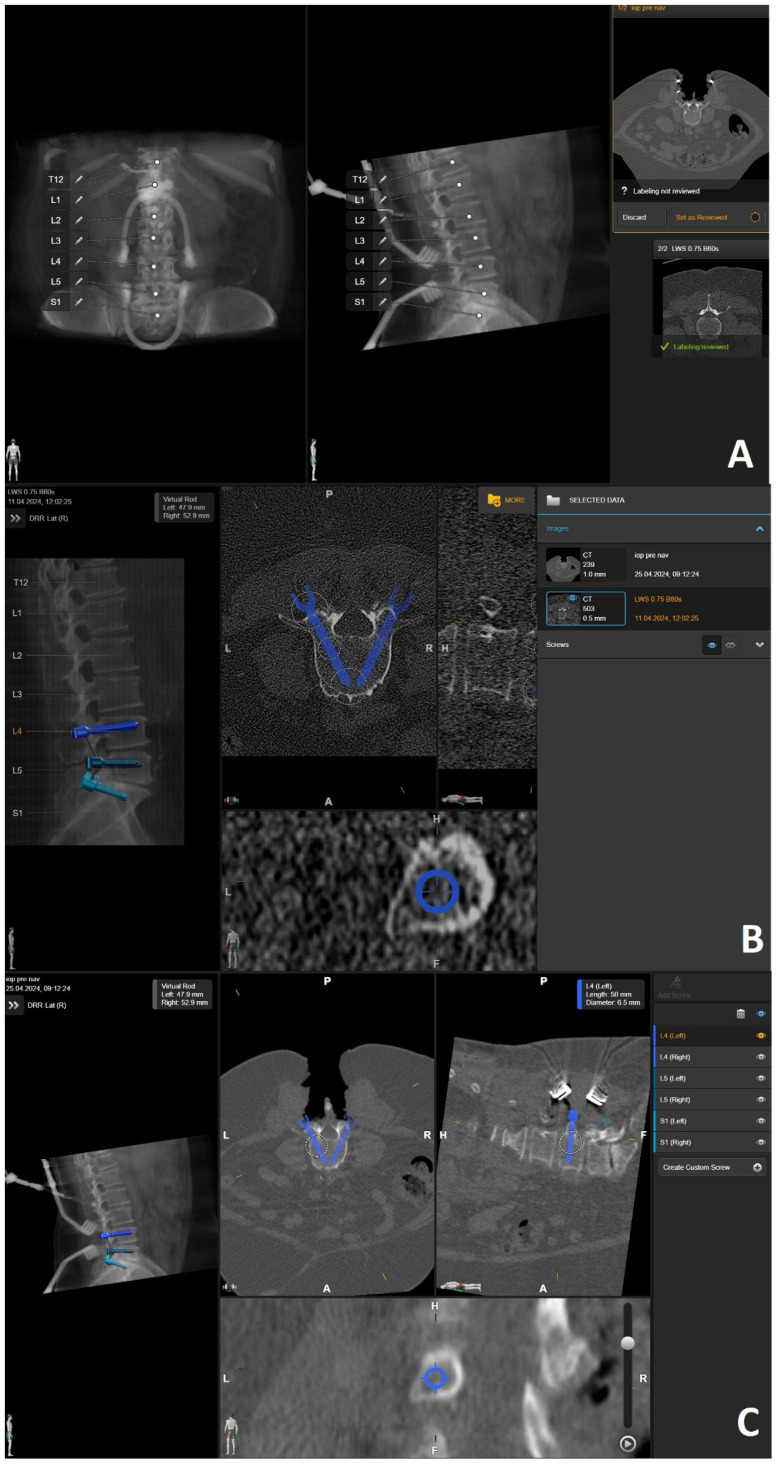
Same patient as in Figure 1, Figure 2 and Figure 7. (A) iCT scan with AI-assisted vertebra recognition. (B) Screw trajectory in the preoperative CT. (C) iCT scan after fusion with preoperative CT shows the screw trajectories, with the option of correcting the screw planning according to the iCT scan.
